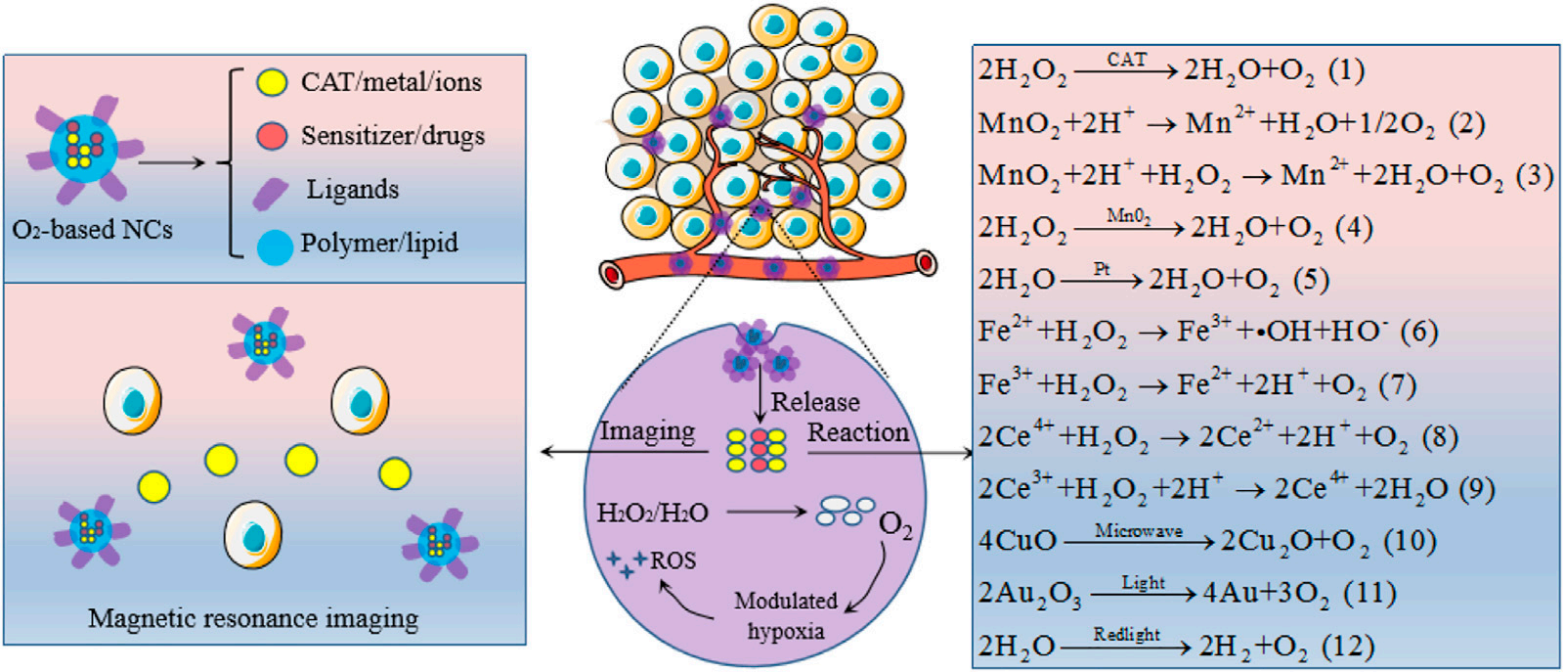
Oxygen-generating NCs to modulate tumor hypoxia and to endow magnetic resonance imaging. The specific mechanisms of oxygen generation are represented by chemical equations.
